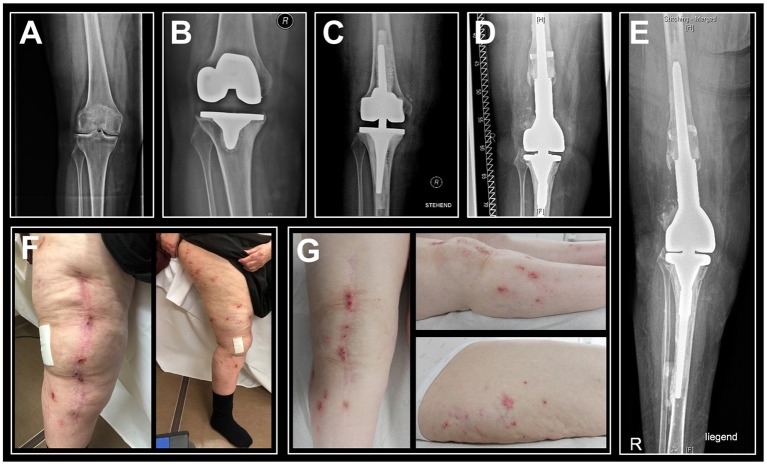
Sequential anteroposterior radiographs of the patient's right knee indicate progression of bone loss and photographs of the right leg show persisting eczema. (A) Radiography prior to primary knee replacement depicts joint degeneration secondary to rheumatoid arthritis, 2009/03. (B) Pre-revisional radiograph indicates peri-implant bone loss and loosening of the tibial component, 2012/03. (C) Post-revisional radiograph depicts intense intraoperative usage of cement and progressive bone loss, 2012/07. (D) Radiographic status at the time of hypersensitivity diagnostics, 03/2018. (E) Latest radiographic status (patient in supine position), 01/2019. (F) Eczema at the time of hypersensitivity diagnostics, 03/2018. (G) Follow-up examination in the aftermath of rituximab therapy indicated an unimproved cutaneous status, 03/2019.
